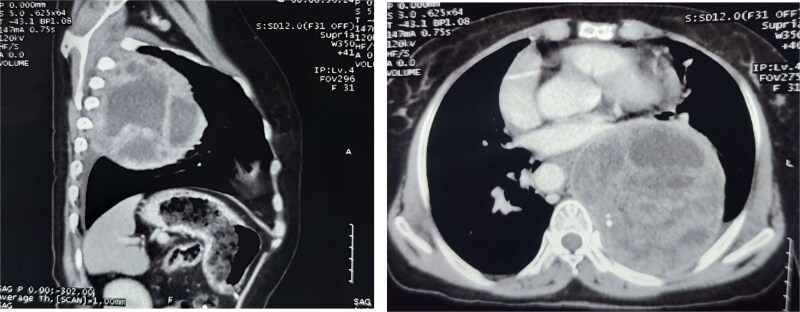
CT chest (axial and sagittal views) showing a large, multiloculated cystic lesion in the left lower lobe, causing compression of adjacent lung parenchyma and mediastinal structures.
